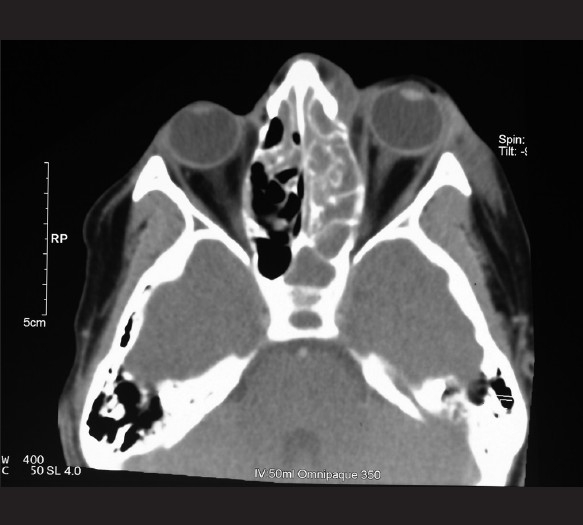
Axial computed tomography scan with contrast showing ethmoid sinus disease and proptosis
